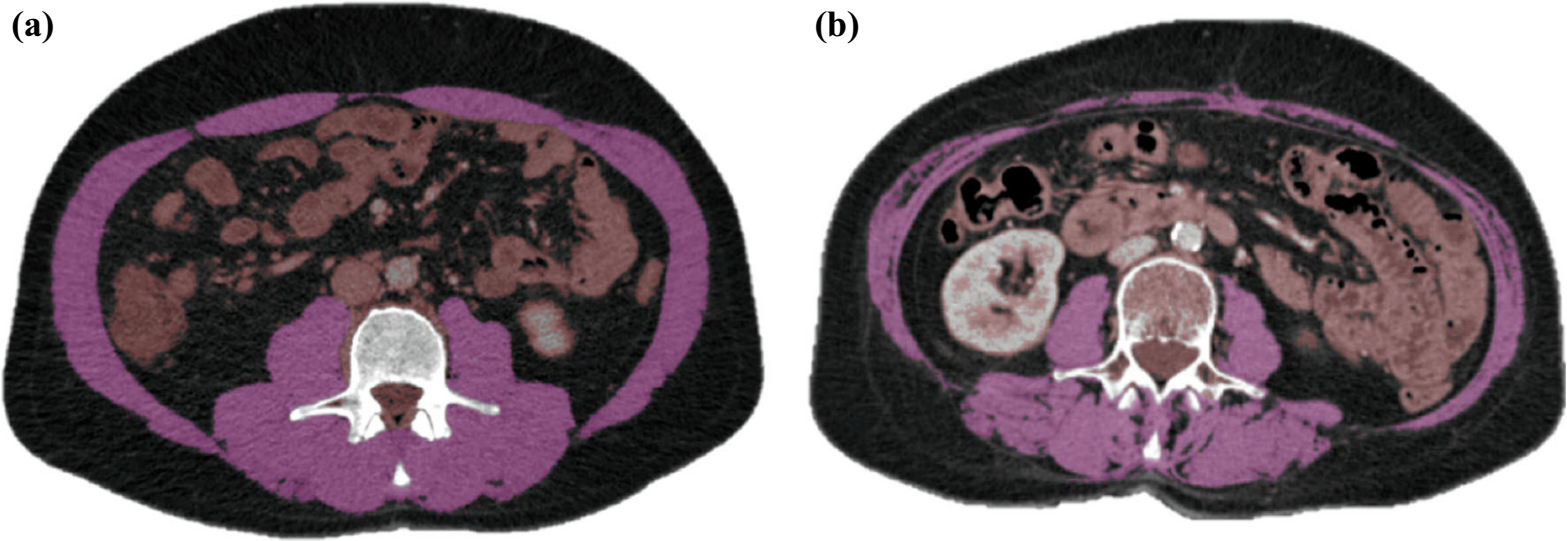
Axial CT slice at the level of the third lumbar vertebra of a male patient with normal SMM (A) and of a female patient with low SMM (B) with peritoneal metastasis from colorectal carcinoma. The psoas, paraspinal, transverse abdominal, external oblique, internal oblique, and rectus abdominis muscles are outlined in *purple* (threshold of − 30 to +150 HU)
